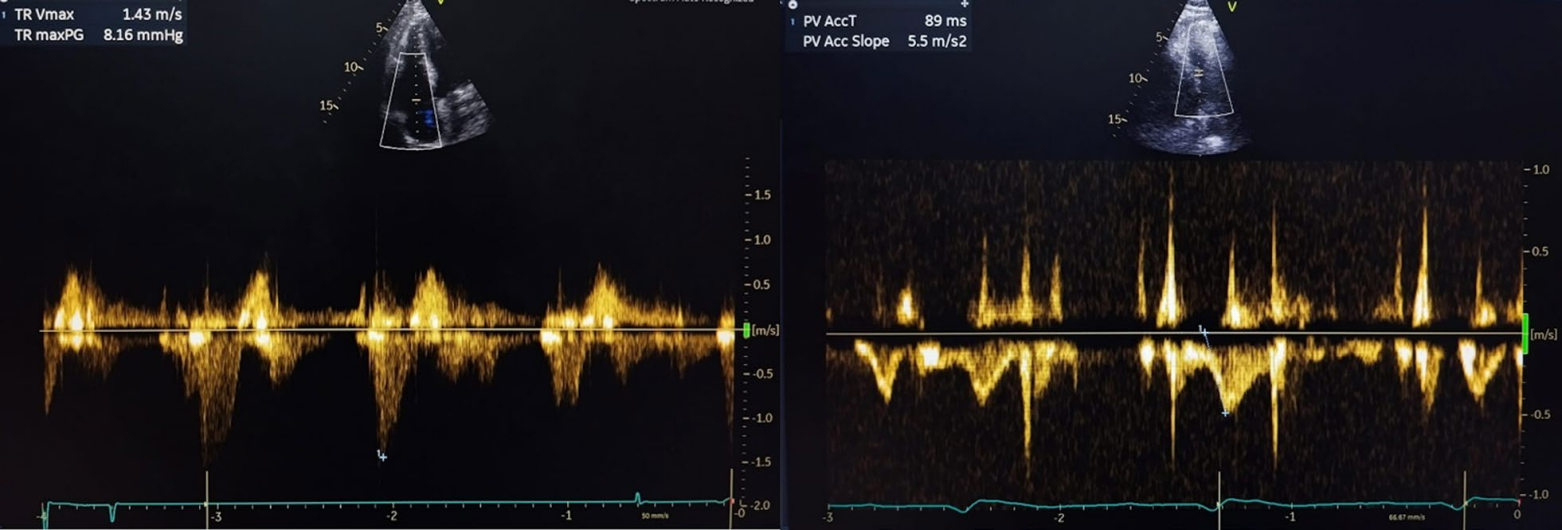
The echocardiography showed tricuspid regurgitation velocity max of 1.43 m/second and pulmonary velocity acceleration time of 89 milliseconds
